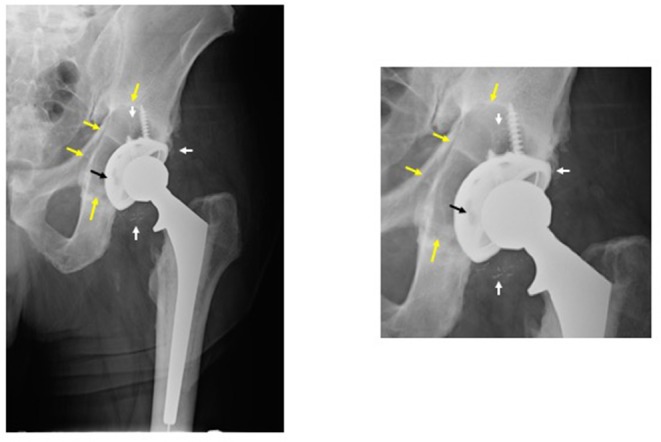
Periprosthetic osteolysis post total hip replacement (THR). The left radiograph shows a hybrid THR with a cemented stem and a cementless cup with screws. The components are well fixed, however, there is polyethylene wear and the metallic cup has fractured adjacent to the screw holes (note: two of the screw holes are larger than they should be and confluent instead of separate—black arrow). The small white radio-dense particles represent metallic debris from the cup (white arrows). There is a large radiolucent black area of bone destruction (osteolysis) (yellow arrows) surrounding the acetabular component. The radiograph on the right is a magnified view of the acetabular area.
